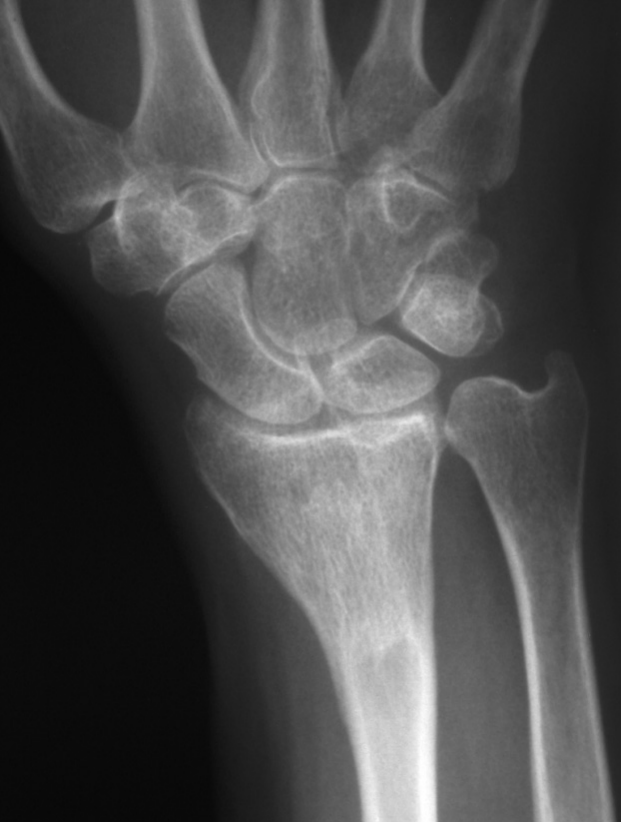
AP radiograph of the same patient one year later with complete incorporation of the graft material and resorption of the graft in soft tissue.
